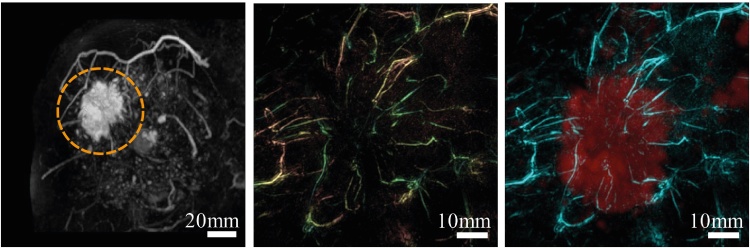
Using the Kyoto-Canon-Optosonics hemispherical imager: the case of a 40-year-old woman with invasive breast cancer, with a 47 mm diameter tumor. First column from left: MR image with lesion marked with red circle. Second column: PA image after eliminating the signals from subcutaneous blood vessels within a depth of 4 mm. Third column: Fusion images of PA (cyan) and MR (red). The PA images demonstrate that tumor associated vasculature converges toward the center of the tumor, tapering drastically at the tumor edge and near the center, where they disappear. (Reproduced with permission of the authors and publisher.)
